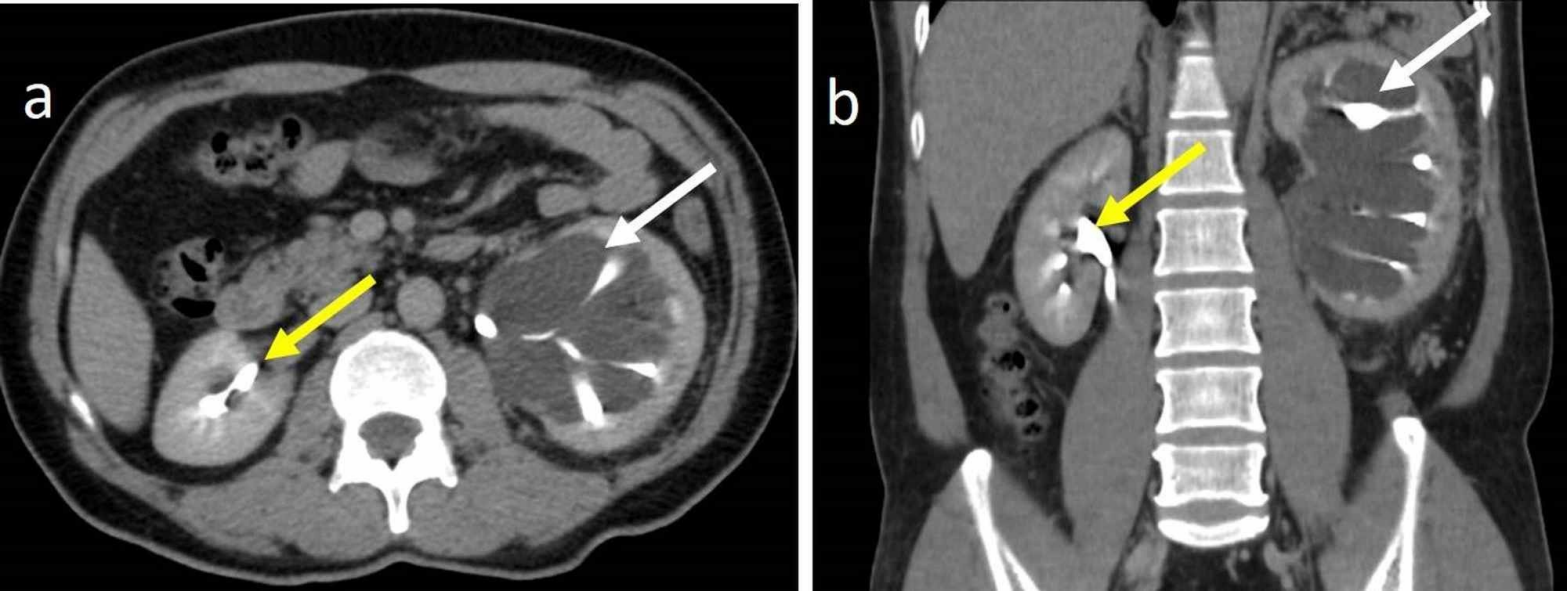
49-year-old male with left peripelvic renal lymphangiectasia (same patient as in Figure 2) Axial (a) and coronal (b) contrast-enhanced CT of the abdomen in the delayed phase demonstrates normal excretion of contrast with splaying of the pelvicalyceal system (white arrow) and non-opacification of peripelvic cystic lesion on the left side. The pelvicalyceal system on the right side (yellow arrow) is normal CT: computed tomography
